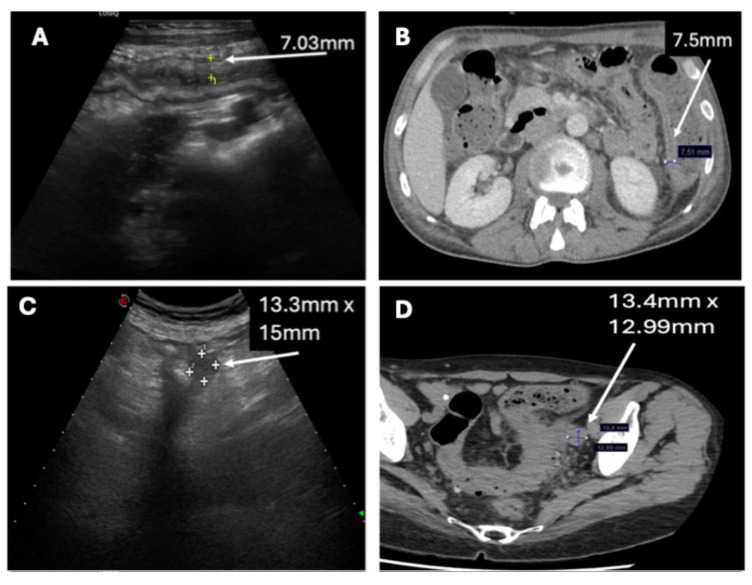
Comparison between CT and BS-US in the diagnosis of NEC (panels A and B) and diverticulitis (panels C and D). (A) BS-US showing NEC with BWT (7.03 mm); (B) CT showing NEC with BWT 7.5 mm. (C) BS-US showing diverticulitis with BWT (13.3 mm × 15 mm); (D) CT showing diverticulitis with BWT (13.4 mm × 12.99 mm).
